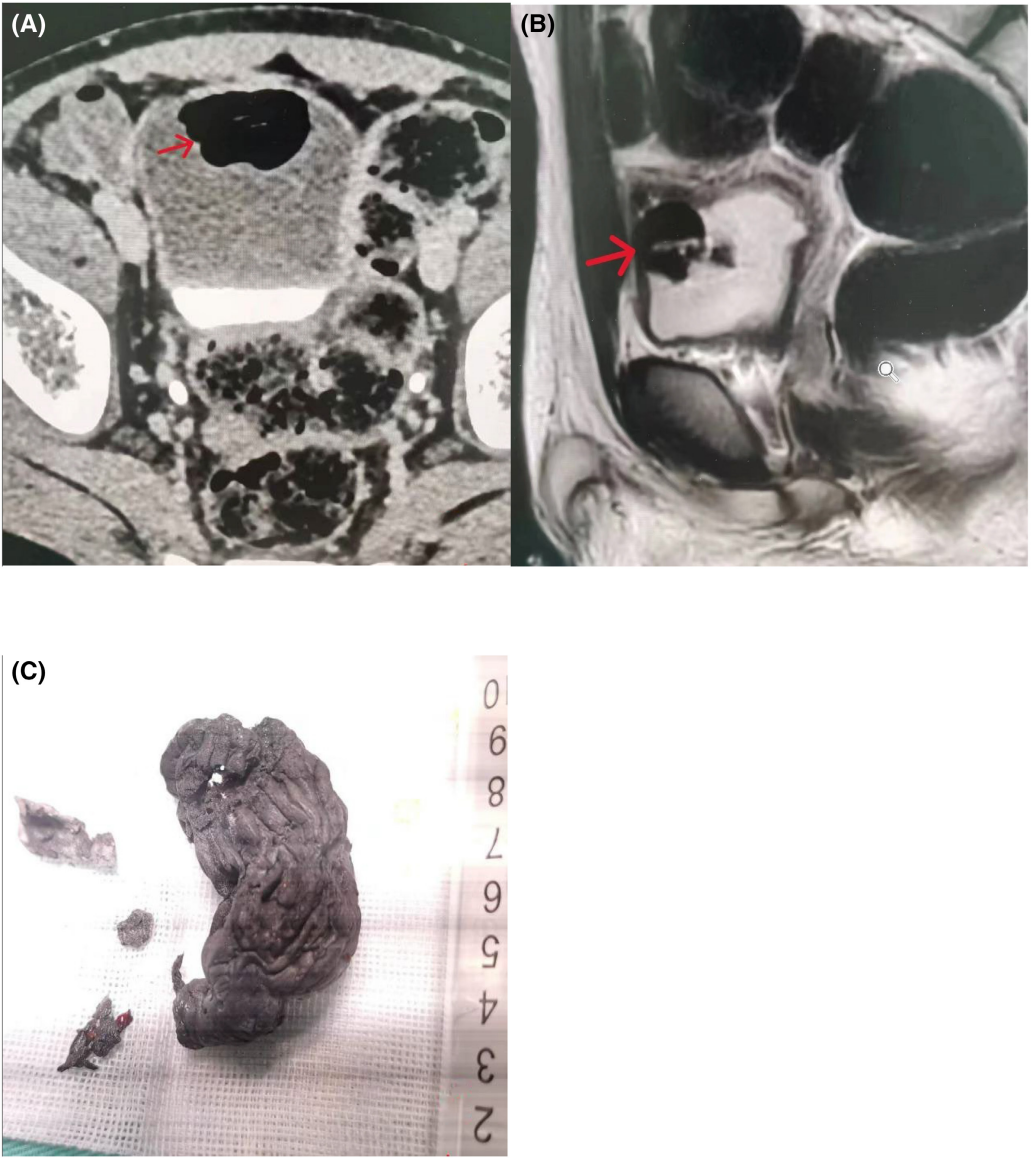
(A) CT showing a lot of air accumulations in the urinary bladder (marked with a red arrow). (B) MRI indicated the air and fluid level in the bladder (red arrow). (C) Retrieved foreign bodies from the urinary bladder.
